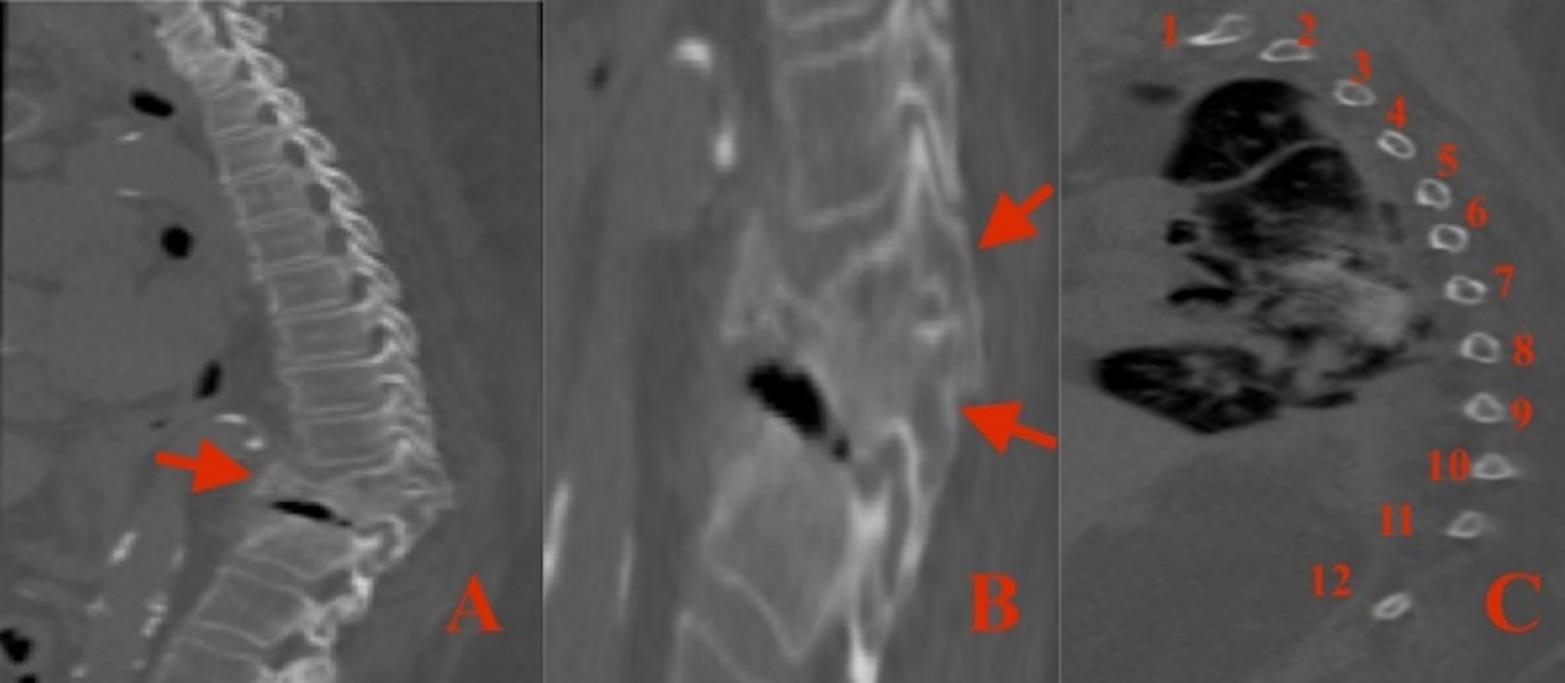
Computed tomography (CT) of the thoracic spine A: Sagittal view demonstrating a T11 burst fracture (arrow) with posterior bony retropulsion, and vacuum disc phenomenon of the T11/T12 intervertebral disc space from advanced degeneration. B: Sagittal view with zoom focused on the T11 vertebral body showing duplication of the vertebral pedicles entering the T11 vertebral body in vertical alignment (arrows). C: Sagittal view utilized for accurate counting of the 12 thoracic ribs, and for verification of their corresponding vertebral body.pedicles entering the T11 vertebral body in vertical alignment (arrows).
